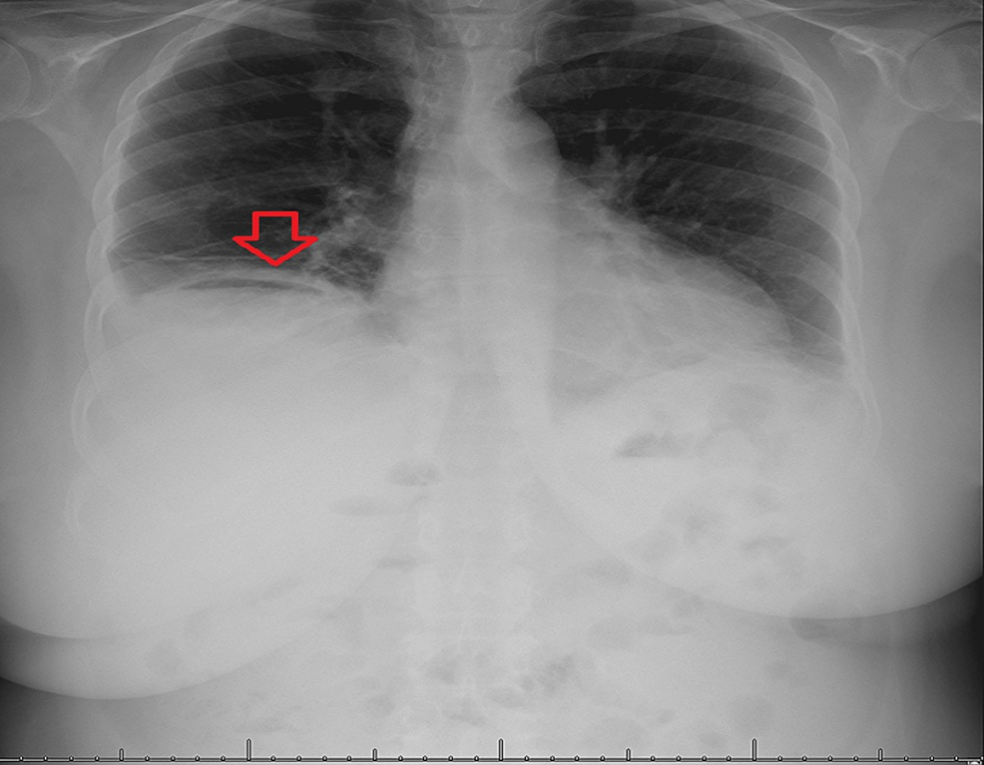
Chest x-ray before discharge revealed minimal residual air
